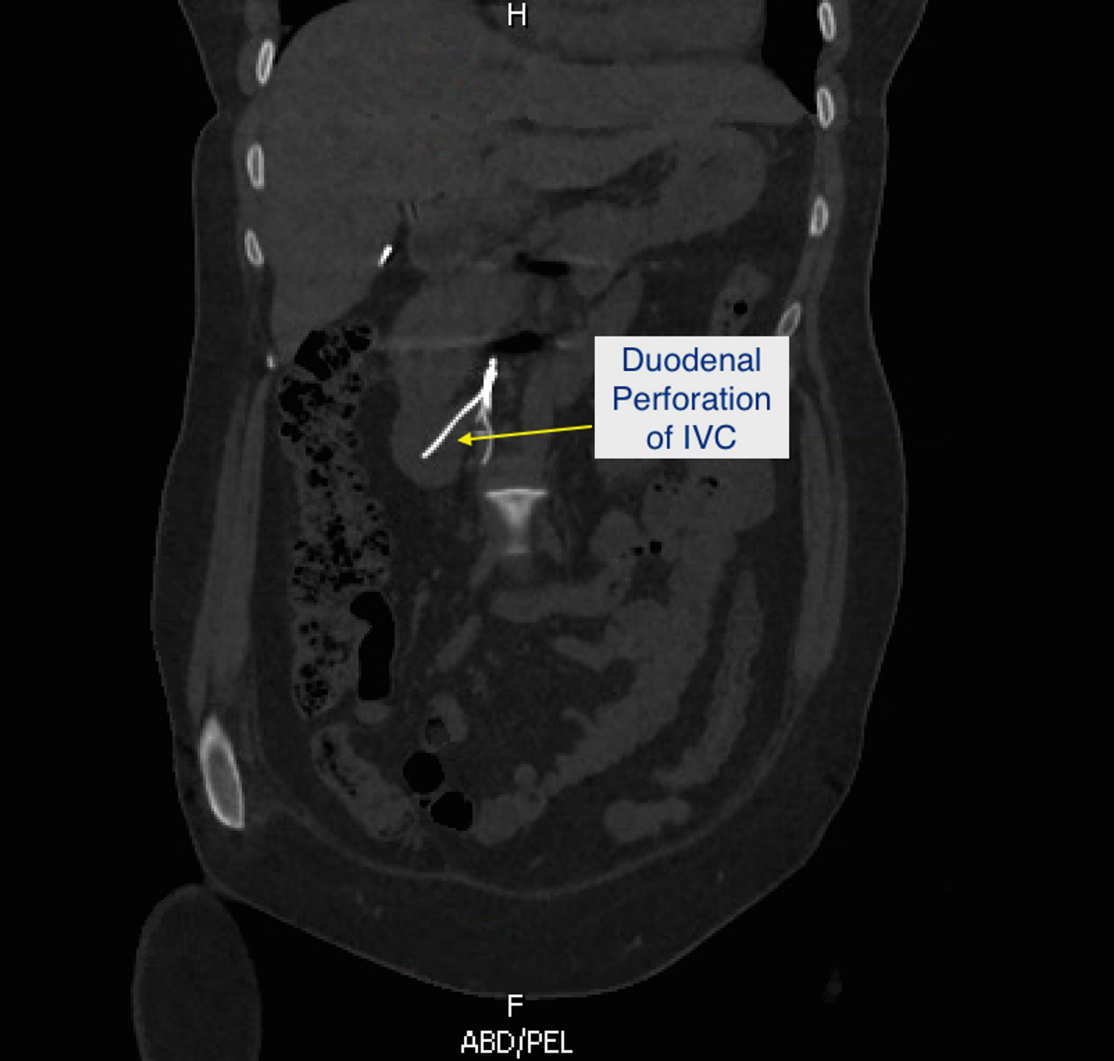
CT scan showing IVC filter penetration into duodenum. CT: computed tomography; IVC: inferior vena cava.
